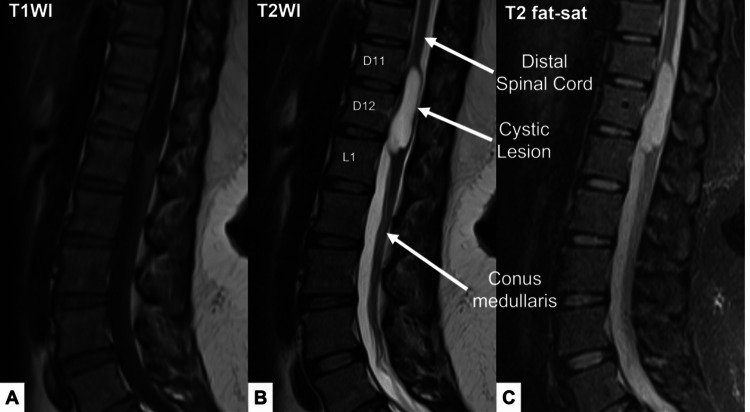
MRI of the patient - image 1 Sagittal and axial imaging with T1-weighted, T2-weighted, and T2 fat-saturated sequences reveal a lesion in the thoracic spine at the level opposite T11/T12 and the upper limit of the L1 vertebral body. The lesion is hypointense on T1 and hyperintense on T2 and T2 fat-saturated images, and it is causing expansion of the thoracic canal and displacement of the spinal cord MRI: magnetic resonance imaging
